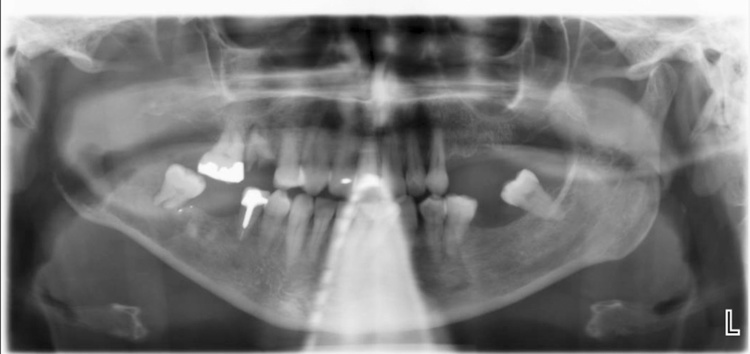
Orthopantomogram (OPG) demonstrating destruction of the right condyle, coronoid and ramus.
